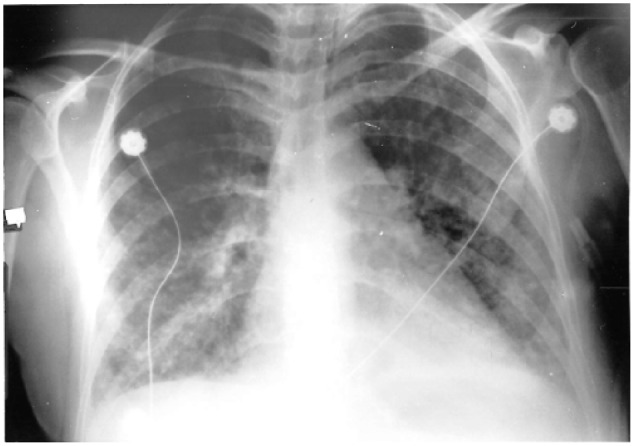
2nd Chest radiograph on hospital admission.
